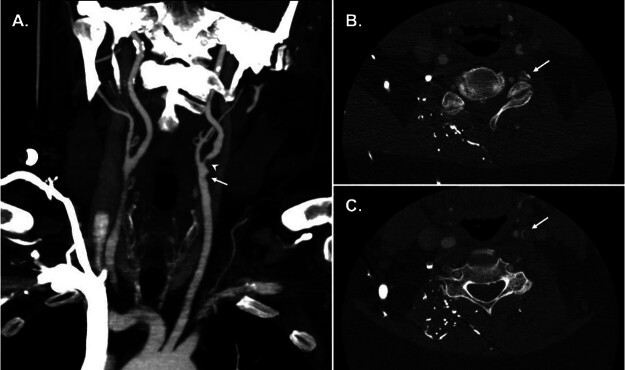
CT angiogram of the neck demonstrating (
